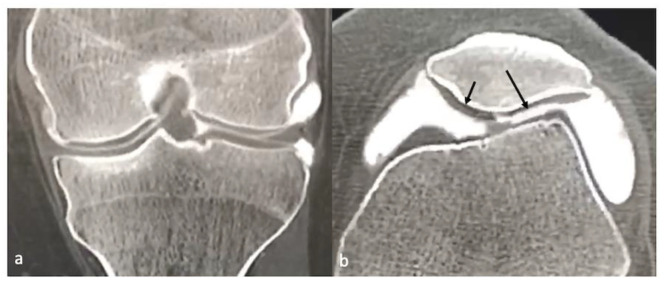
CT arthrogram of the knee coronal (a) and axial (b) planes showing normal cartilage (small arrow) and focus of partial thickness cartilage defect (long arrow).
